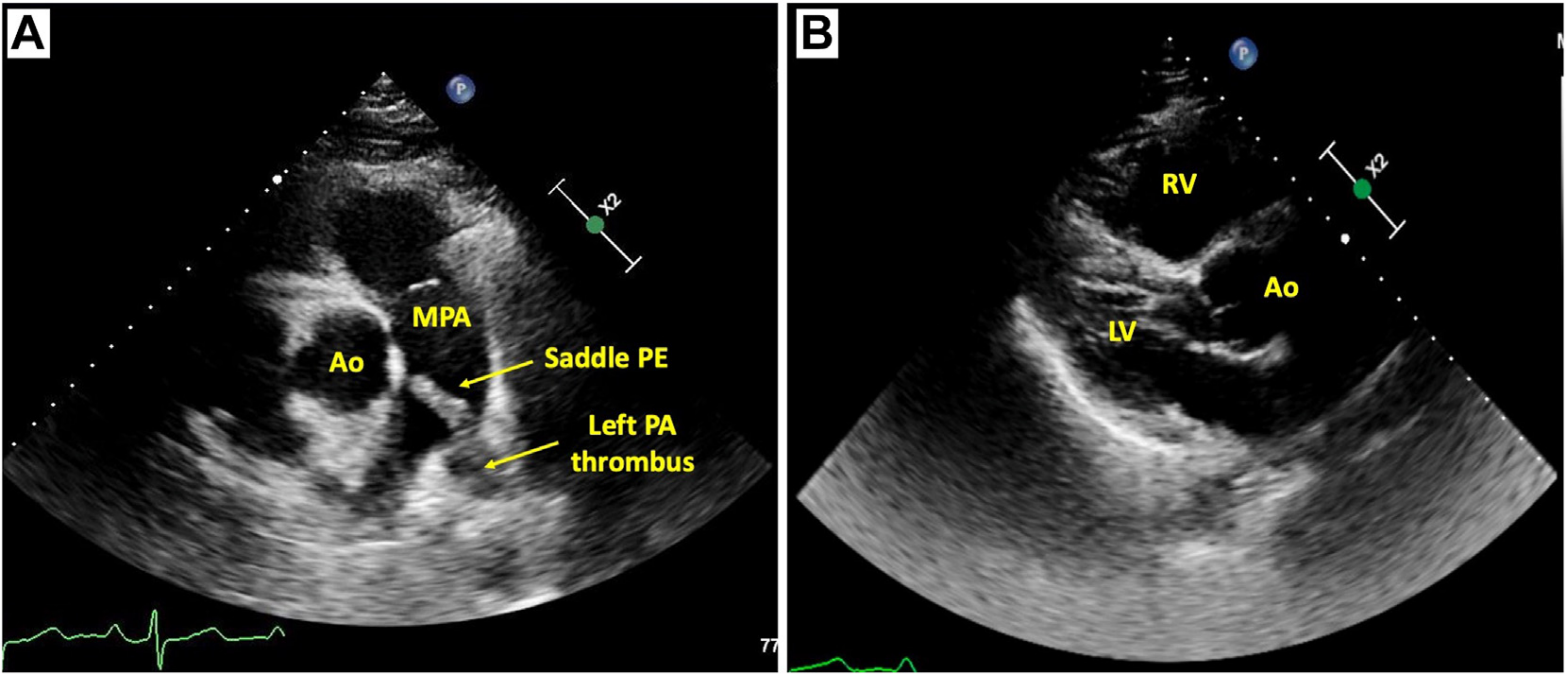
Transthoracic echocardiogram: saddle pulmonary embolism. Parasternal short-axis view of the bifurcation of the PA in diastole. Left PA PE extending to the main PA is demonstrated (A). Parasternal long-axis view in diastole demonstrating RV dilatation (proximal RV outflow tract diameter, 4.0 cm; normal range, 2.1-3.5 cm) (B). *Ao*, Aorta; *MPA*, main PA.
